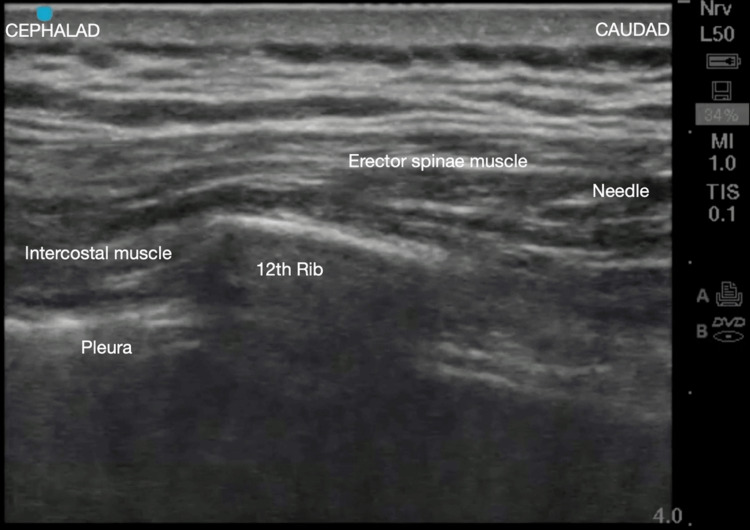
An ultrasound image of the cryoanalgesia needle in the vicinity of the 12th rib and the pleura.
